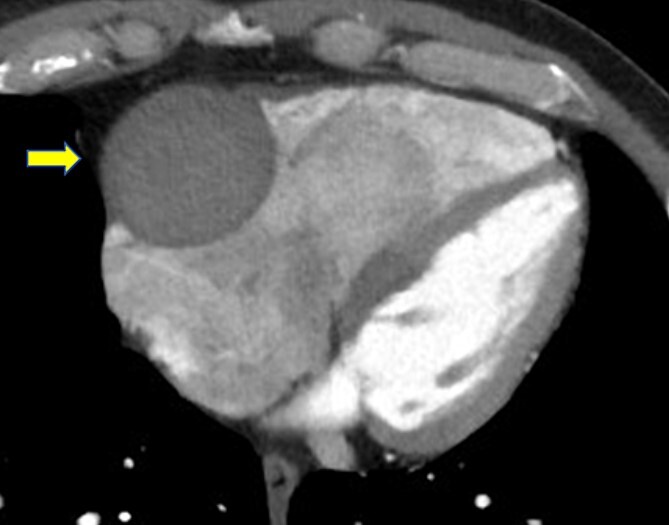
Enhanced computed tomography shows a giant coronary artery aneurysm (arrow) at the right coronary artery, which is occluded completely by a thrombus.
